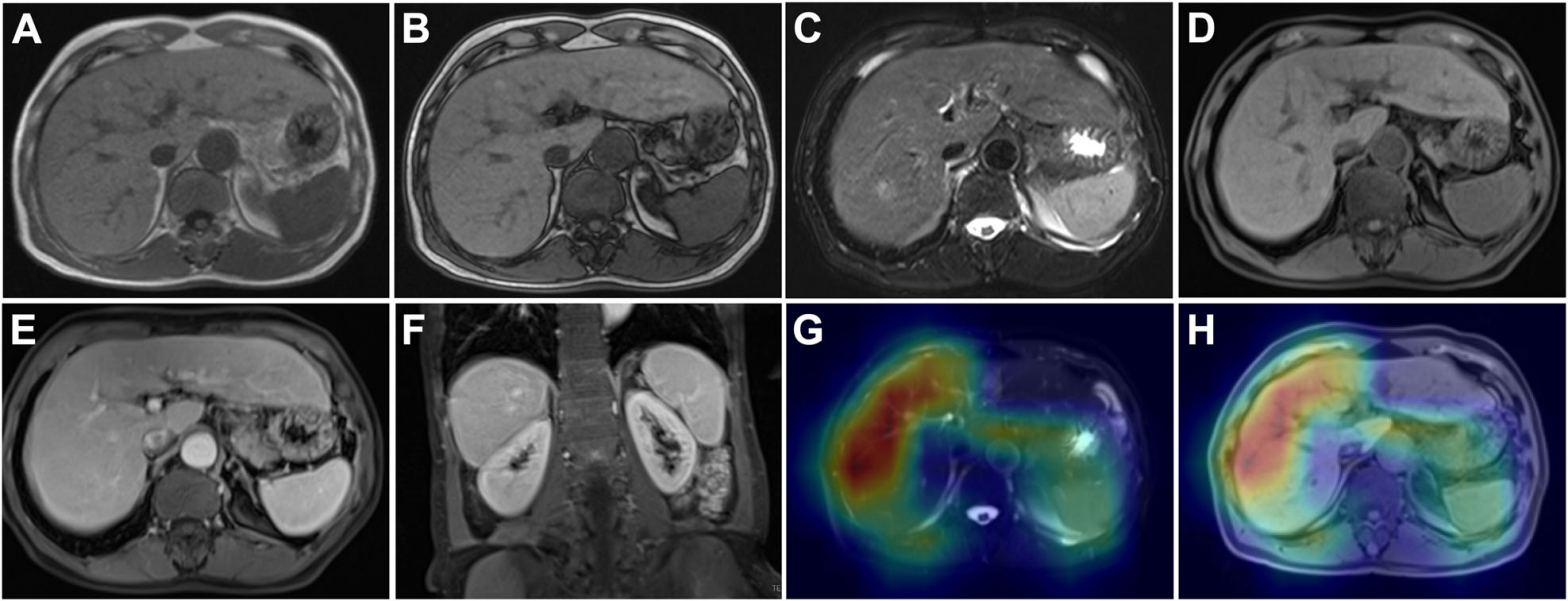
Extracellular contrast agent-enhanced MRI of a 67-year-old male patient with cirrhosis and chronic hepatitis B. In- and opposed-phase images (A, B), T2-weighted image (C), pre-contrast T1-weighted image (D), and portal venous phase images (E, F) revealed no surface nodularity or other morphological changes and no manifestations of portal hypertension. Activation maps overlaid on T2-weighted (G) and pre-contrast T1-weighted (H) images showed highlights in liver parenchyma, liver surface, and spleen. The combined model- and CNN model-based cirrhosis probability were 0.77 and 0.64, respectively, which both lead to a diagnosis of cirrhosis. Neither radiologist diagnosed this case as cirrhosis
